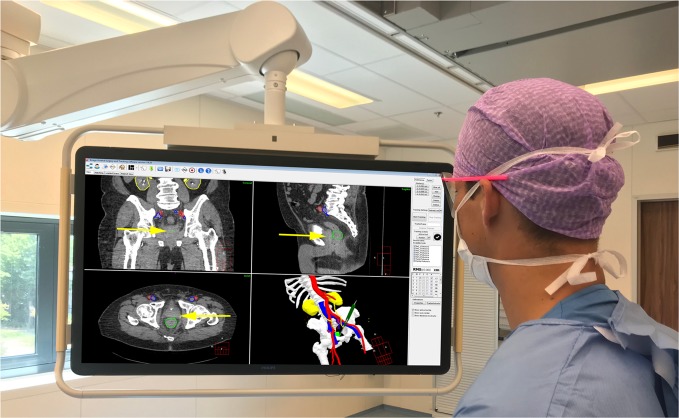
Surgical navigation user interface during surgery showing the planning CT with segmentations (top-left corner: coronal view; top-right corner: sagittal view; lower-left corner: axial view) and 3D model (lower-right corner). Visible segmentations: bones (white), arteries (red), veins (blue), ureters/kidneys (yellow), and tumor (green). The visible slice of the CT scans is based on the location of the tip of the surgical pointer which is highlighted with yellow arrows.
